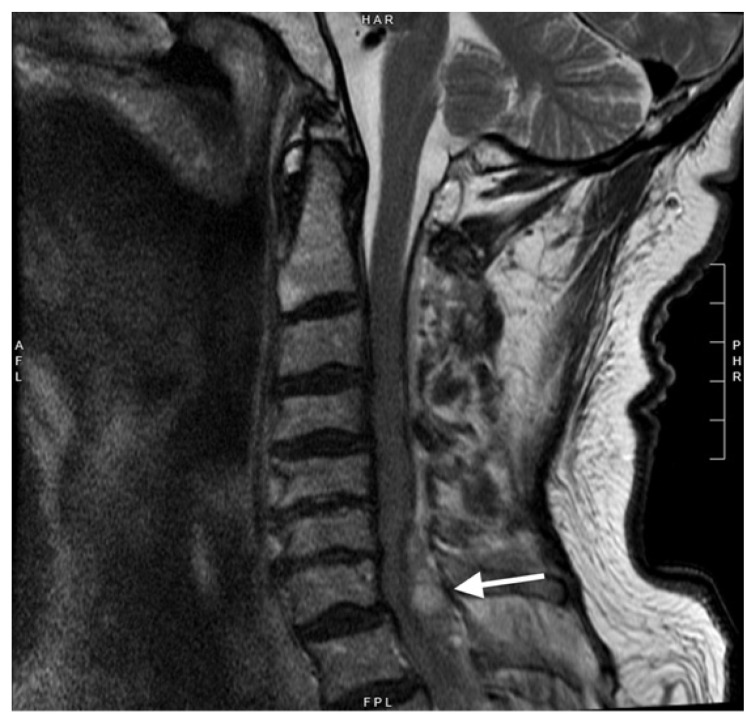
Sagittal T2-weighted, non-contrast magnetic resonance imaging of the cervical spine showing hyperintense dorsal epidural hematoma with cord compression most pronounced at the seventh cervical vertebra (arrow).
